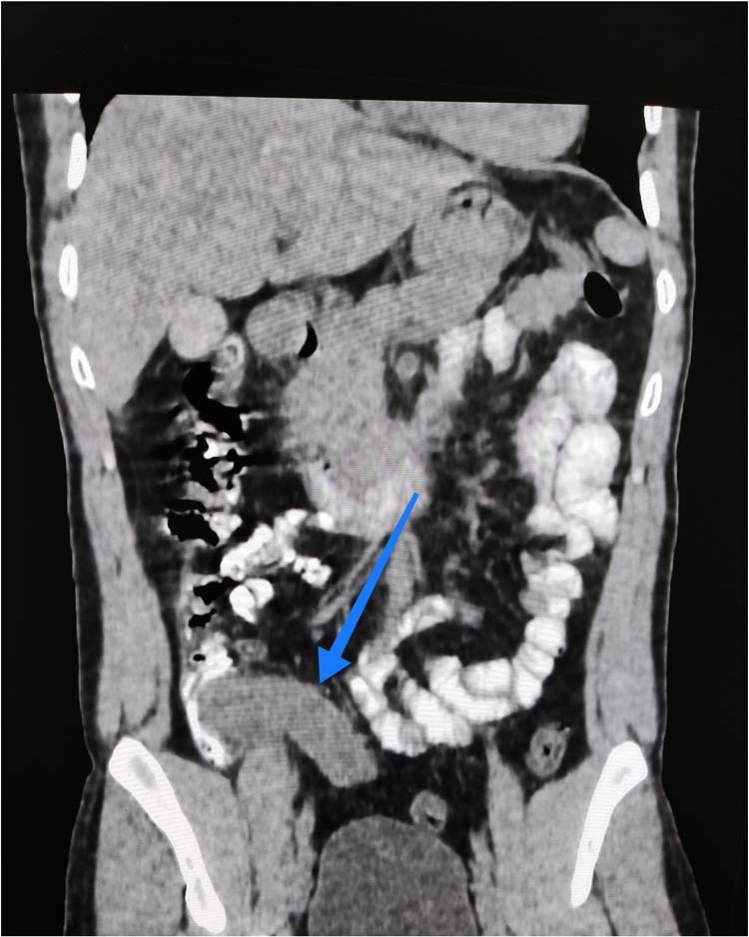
Coronal view of abdominal contrast CT showing the oblong structure originating from the medial side of the caecum and extending down to the pelvis.
